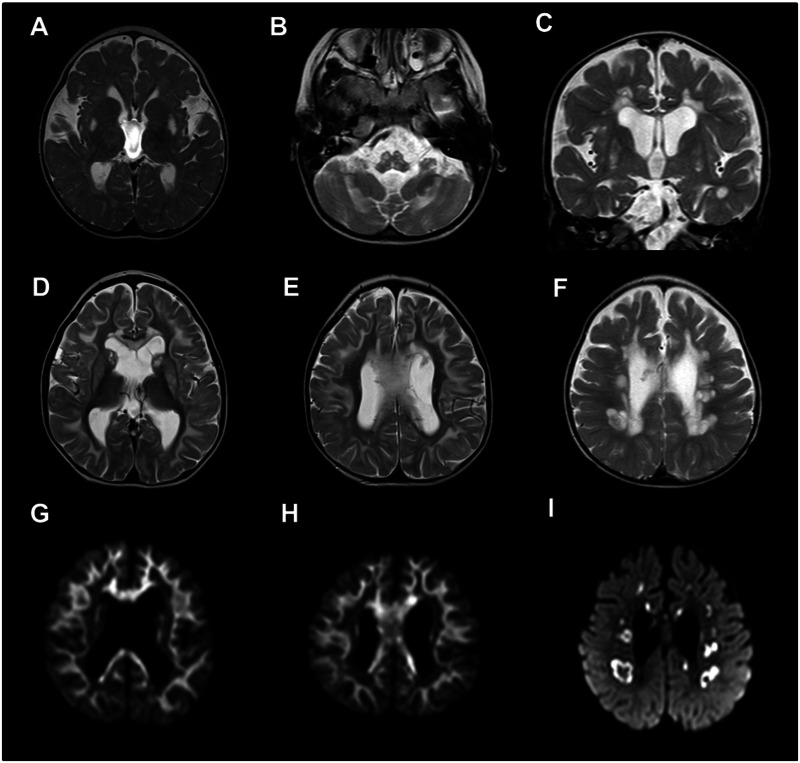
Brain abnormalities in patients harbouring *LRPPRC* mutations identified by cranial magnetic resonance imaging. (A) Axial T_2_ demonstrating symmetrical T_2_ hyperintensity in the putamina (Patient 8). (B) Axial T_2_ demonstrating symmetrical medullary and cerebellar hyperintensity (Patient 8). (C) Coronal T_2_ demonstrating symmetrical putaminal and periventricular T_2_ hyperintensity (Patient 10). Appearances in A–C are consistent with Leigh syndrome phenotype. (D–F) Axial T_2_ images of the brain with corresponding diffusion-weighted imaging sequences (G–I) demonstrating active demyelinating leucoencephalopathy involving the subcortical U-fibres, basal ganglia and corpus callosum in D, E, G and H (Patient 2) and cystic demyelination in the deep white matter in F and I (Patient 8).
